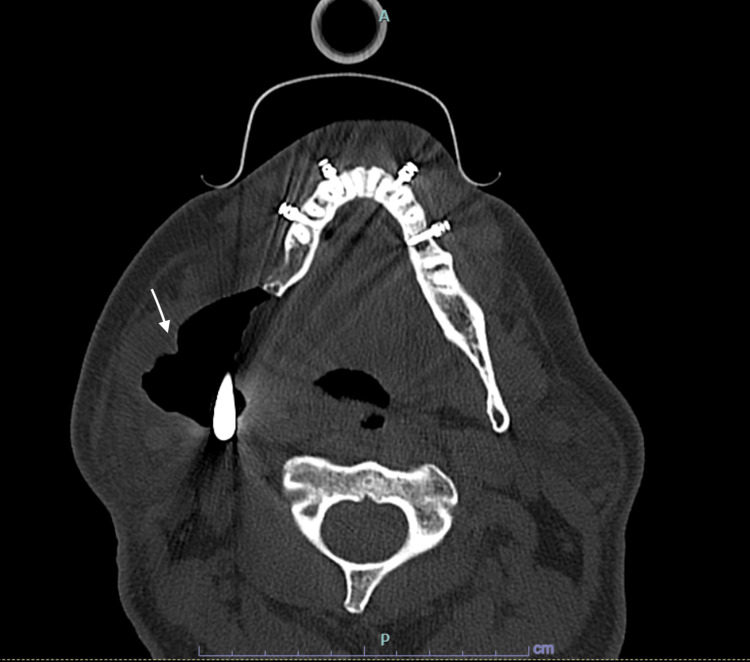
Axial CT scan of facial bones without contrast status post right mandible tumor resection and TMJ reconstruction Axial CT scan with bone window setting demonstrates an asymmetric enlargement of the right pterygoid and masseter muscles. A large amount of air is noted adjacent to the right mandibular prosthesis (arrow).
